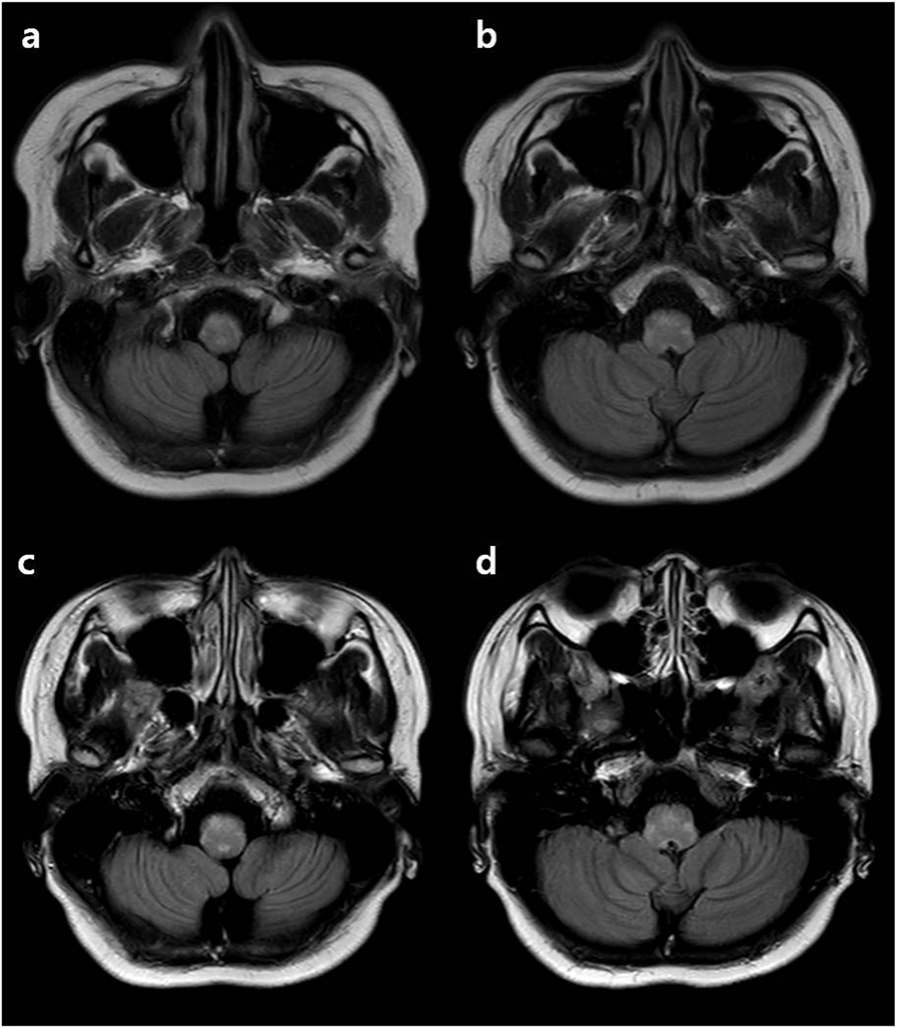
a, b, c, d Brain MRI taken at 1 week after admission (a, b) Fluid attenuated inversion recovery (FLAIR) MRI showed high signal lesions in the medulla oblongata. (c, d) FLAIR with contrast enhancement MRI showed mild enhancement in the same area
